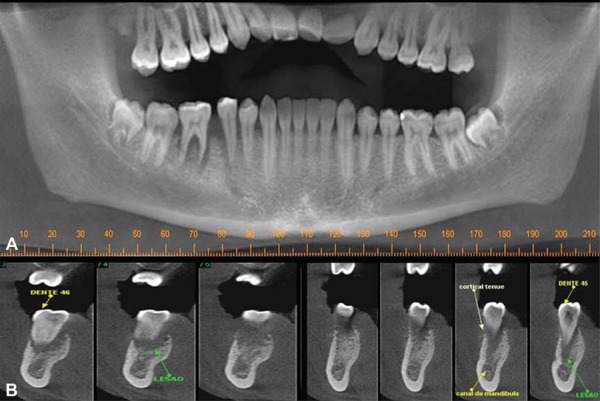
Cone beam computed tomography (A–B) showing a well-defined osteolytic lesion associated with the roots of teeth #29 and #30. Cross-sectional image (B) allowed observing alterations in the trabecular bone
